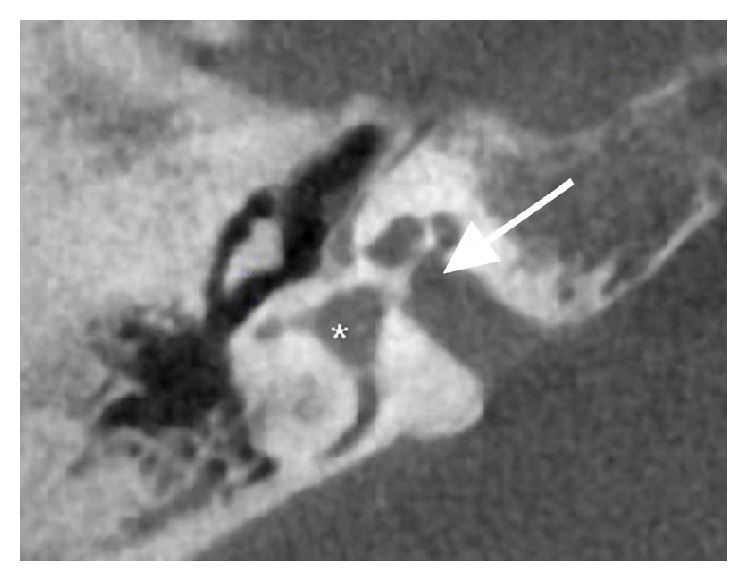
Axial noncontrast computed tomography of right temporal bone reveals incomplete separation of the right internal auditory canal from the basal turn of the cochlea (arrow) and an enlarged vestibule (asterisk).
